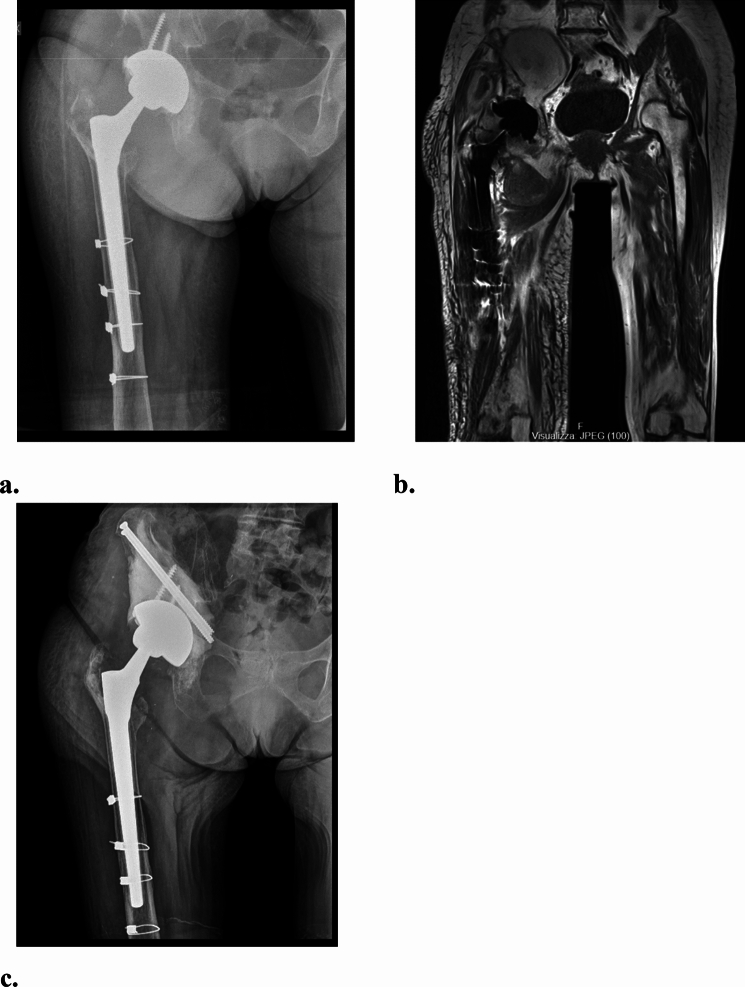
a–c An 84-year-old female presented with soft tissue swelling and pain, showing a large intrapelvic pseudotumor and normal blood metal ion levels. The stable Metal-on-Polyethylene cup and stem classified the case as Psi C1 S1 (PCS). Treatment involved preoperative embolization, mass debulking, and replacing the head and liner with ceramic and polyethylene. Despite an ischial bone defect, cup stability remained intact. The defect was filled with cement and secured with screws. a Preoperative anteroposterior view of the right hip; b T1w-MARS MRI, coronal view showing large intrapelvic and extrapelvic lesions; c Postoperative anteroposterior x-ray of the right hip
